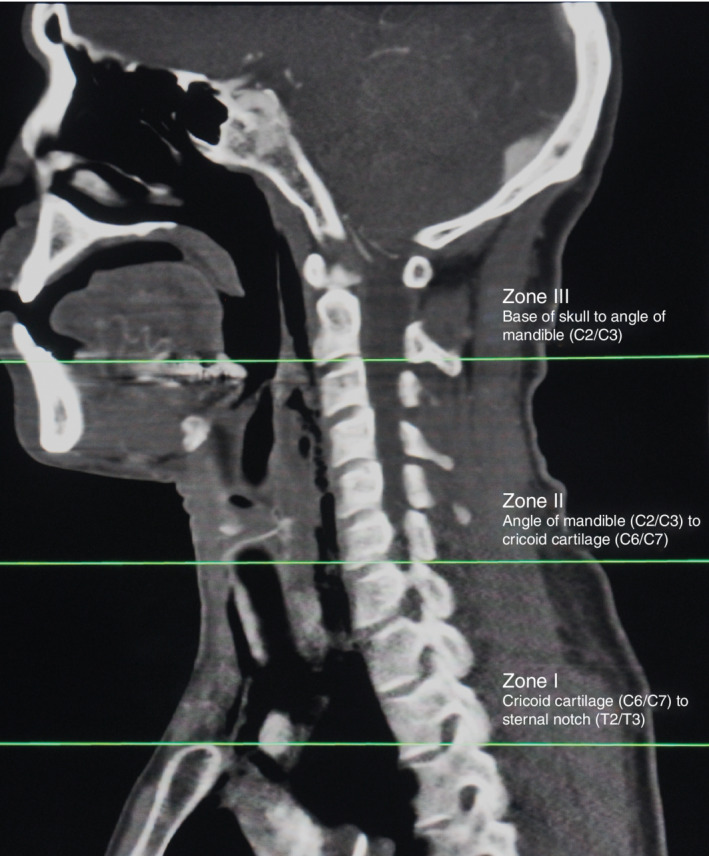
Roon and Christensen's classification^4^ of neck zones as seen on sagittal cervical CT angiography
